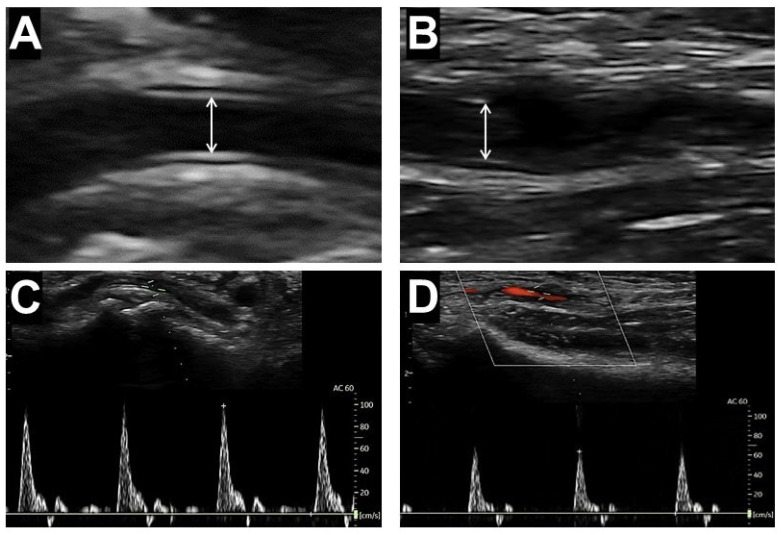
Ultrasound measurement of lumen diameters and blood flow velocities in the distal radial artery (A,C) and forearm radial artery (B,D). Arrows indicate the luminal diameter of the artery, measured as the distance between the intimal layers of the intima-media complex.
